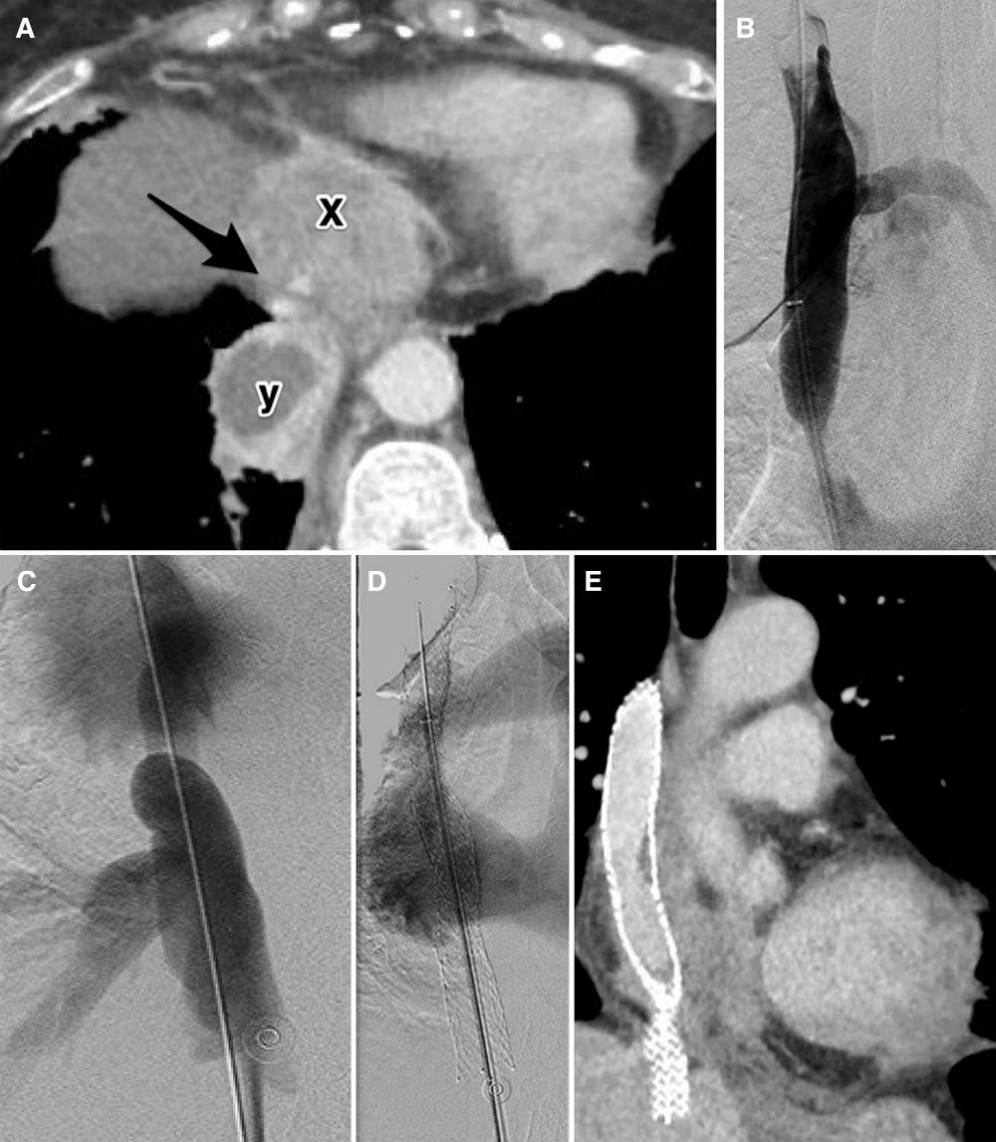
(*A*) Female in her 70 s presenting with IVC syndrome, ultimately diagnosed with IgG4 fibrosing disease (patient 2). Axial image of a contrast-enhanced CT chest shows a pericardial mass (x) causing severe stenosis of the suprahepatic IVC (arrow). Note is made of the known intrapulmonary sequestration (y). (*B*) Superior vena cavagram shows severe stenosis of the superior cavo-atrial junction due to extrinsic compression from a pericardial mass. (*C*) Inferior vena cavagram shows severe stenosis of the IVC above the confluence of the hepatic veins. The hepatic IVC is distended with reflux of contrast into dilated hepatic veins. (*D*) Venogram post stent deployment shows relief of SVC stenosis with flow through the SVC-to-IVC bridging stent to the right atrium. (*E*) CT chest 29 months post stent deployment shows a patent SVC-to-IVC bridging stent.
